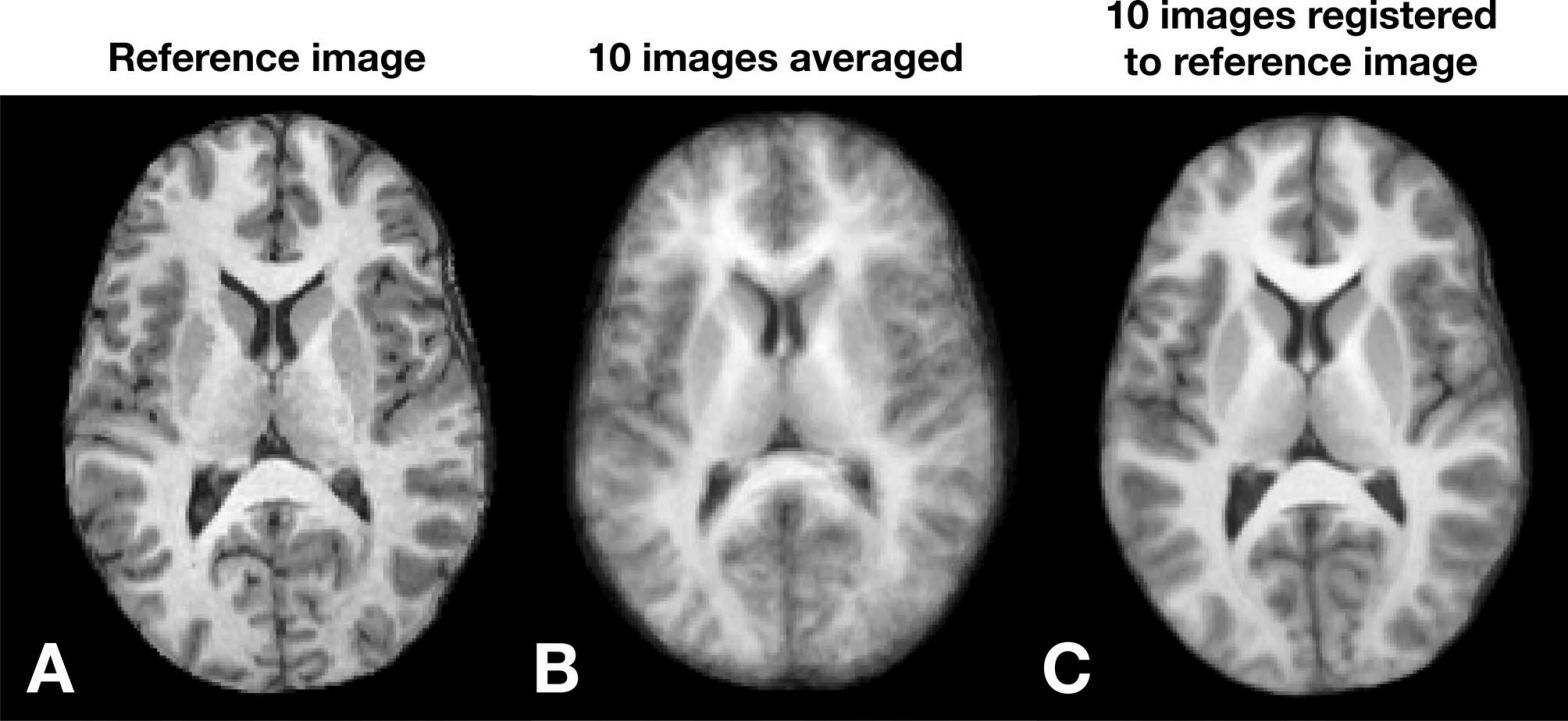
Registration of multiple high resolution anatomical (HRA) MRI scans using symplectomorphic registration (SYMREG). (A) Single reference HRA, (B) average of HRA datasets from 10 different people. Blurring is do to subtle natural shape variations between individual subjects (C) average of 10 images in (B) after registration with template image in (A) from (adapted from^43^).
